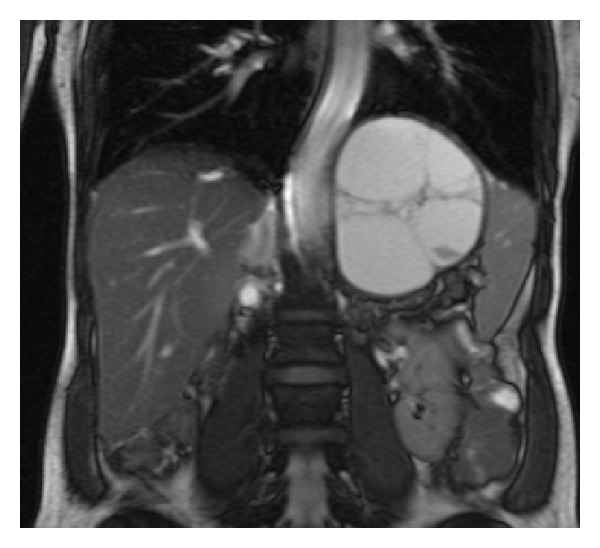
High signal on T2-weighted magnetic resonance imaging.
